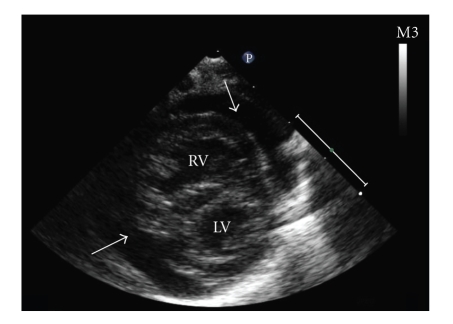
Echocardiogram on day 1 of admission showing massive pericardial effusion present globally around the heart.
